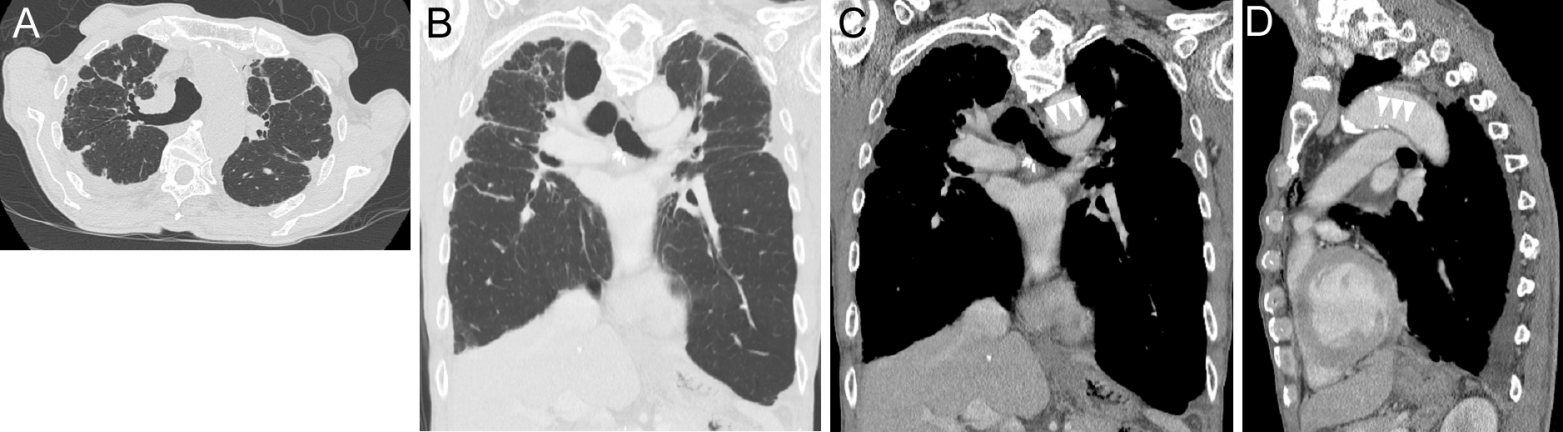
CT of the chest, showing bilateral subpleural predominant consolidation with traction bronchiectasis and volume loss. The lesion was predominant in the right lung, and cysts and traction bronchiectasis were widely observed in the right upper lobe. The volume loss of the right upper lobe was remarkable, and a rightward mediastinal shift was noted (A, transverse view of high-resolution CT on initial consultation; B, coronal view responding to C at the onset of hoarseness 2 months after the initial consultation). The aortic arch and left pulmonary artery were closely contacted, possibly compressing the left recurrent laryngeal nerve (white arrowheads in C, coronal view, and in D, sagittal view at the onset of hoarseness).
